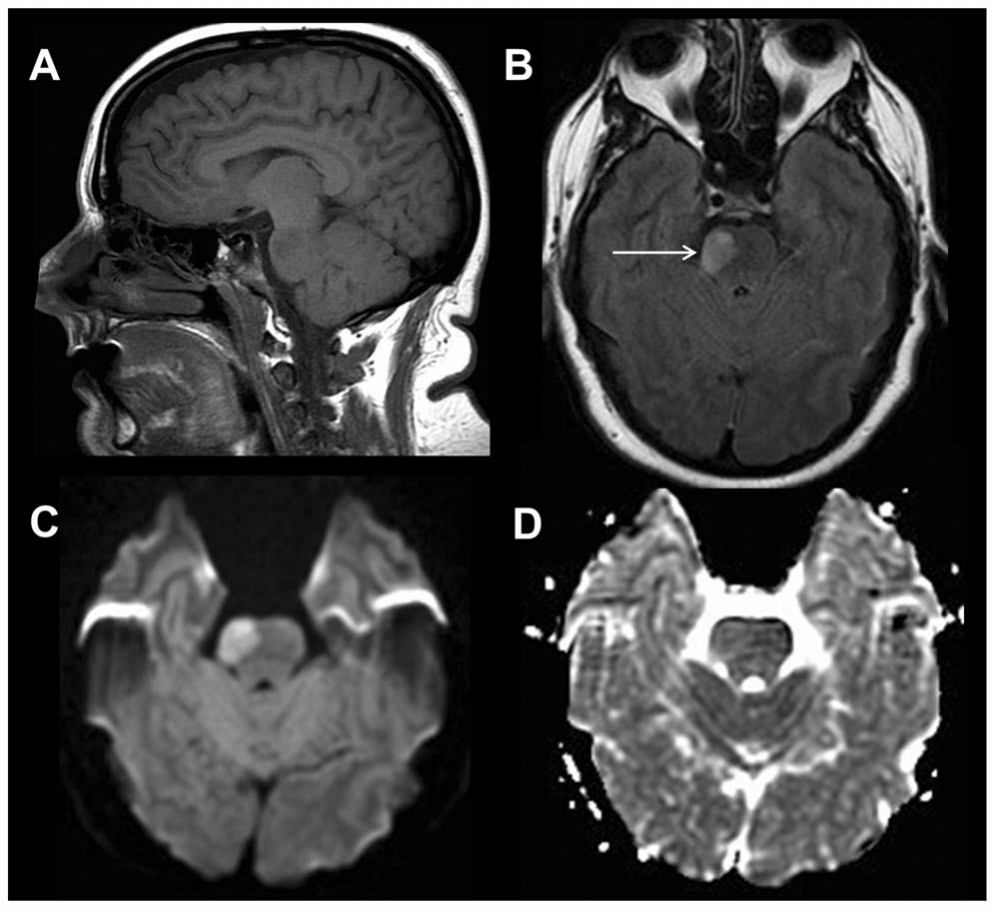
Brainstem mass lesion. 38 year old female with transient left sided weakness. Sagittal T1-weighted image (A) shows a subtle hypointense lesion within the pons. Axial T2-weighted FLAIR image (B) demonstrates a hyperintense, expansile mass-like lesion within the right mid pons (white arrow). Axial diffusion weighted trace (C) and apparent diffusion coefficient images (D) reveal slightly increased diffusivity within the lesion compatible with a demyelinating lesion, neoplasm, or subacute infarction.
